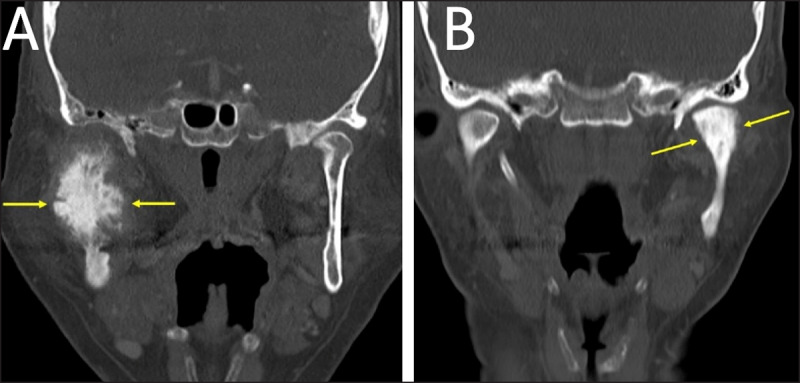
(A, B) Sclerotic mandibular metastases. Coronal CT obtained in two different patients showing metastases of prostate cancer (a) and breast cancer (b) (arrows).
